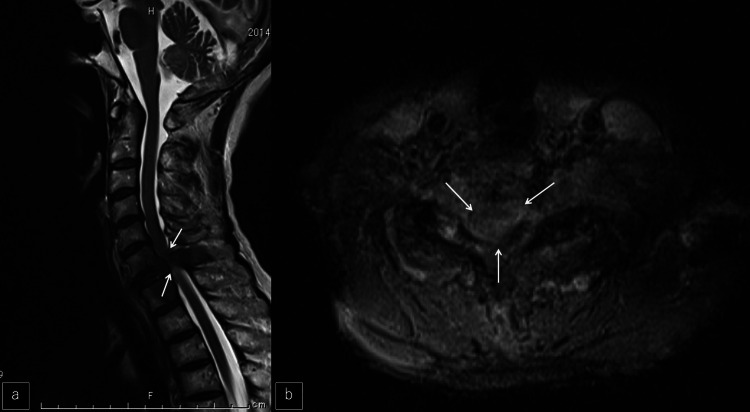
MR imaging at the first visit a, b: Sagittal and axial MRI. A severely compressed spinal cord was shown at the C7 vertebra (white arrows).
